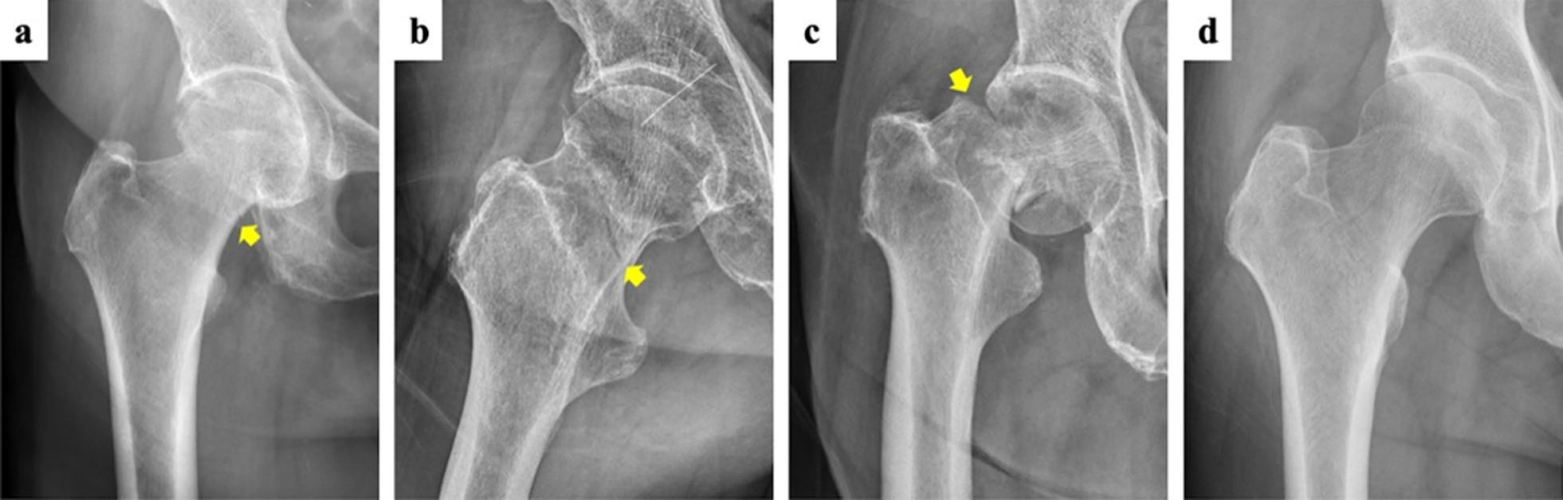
Sample X-ray images. Images of varying levels of difficulty, as judged by our expert radiologist and orthopediatrician. There were three levels of difficulty: difficult (level III, a), medium (level II, b), easy (level I, c), and healthy images as the control (d). Arrow indicates the fracture line (not shown to the participants)
